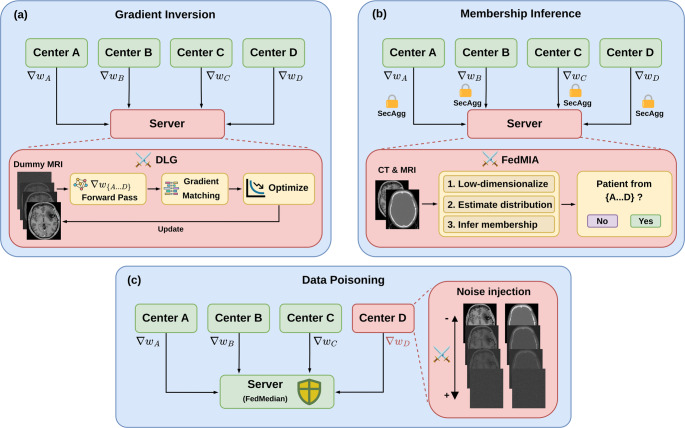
Overview of the three attack scenarios and corresponding defenses evaluated in the proposed federated sCT synthesis framework. *(a) Gradient Inversion:* a honest-but-curious server attempts to reconstruct private MRI training data from the gradients $$\nabla w_{\{A\ldots D\}}$$ via the DLG attack, which iteratively optimizes a dummy input through gradient matching. *(b) Membership Inference:* the FedMIA attack exploits client gradients to determine whether a given CT–MRI pair was included in the training set of any participating center; SecAgg is adopted as a countermeasure, encrypting each client’s gradient prior to server-side aggregation. *(c) Data Poisoning:* a malicious client corrupts the federation by injecting additive noise into its local training data, thereby altering the contributed gradient; FedMedian aggregation is employed at the server as a robust defense against such poisoned updates
